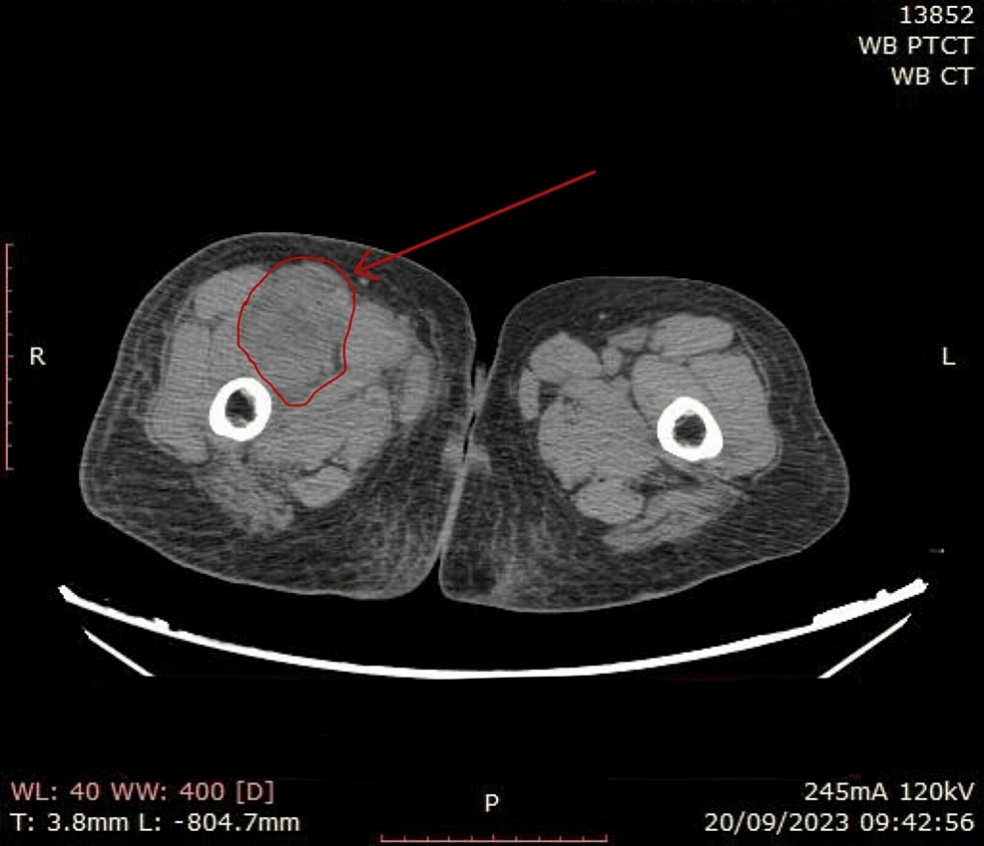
Whole-body PET-CT scan CT image showing a soft tissue mass in the right anterior compartment of the thigh.
